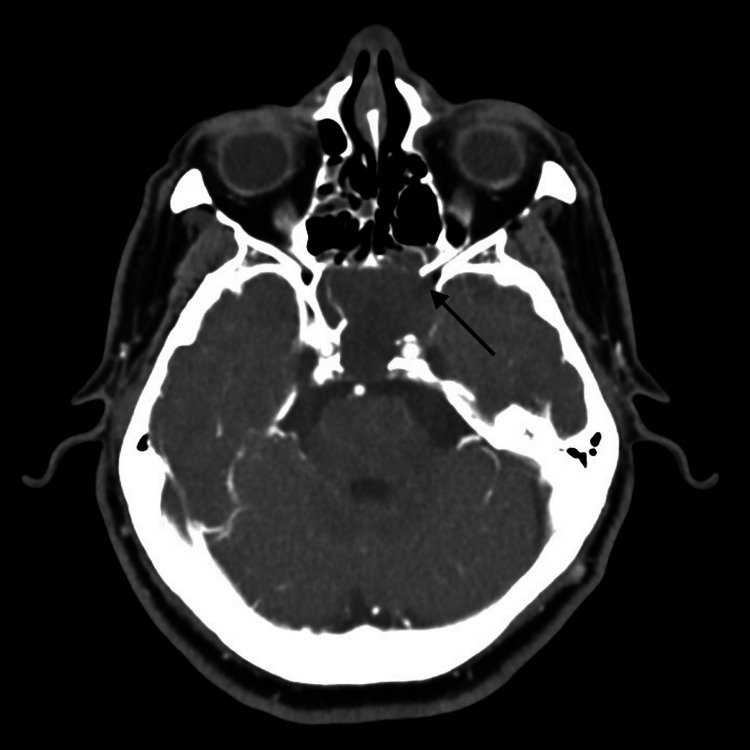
CT scan of the paranasal sinuses with contrast The image shows a heterogenous hypoattenuating mass in the sphenoid sinus, causing erosion of the roof, floor and lateral walls of the sphenoid sinus and encroaching on the ipsilateral optic canal and the superior orbital fissures of the left orbit (black arrow).
